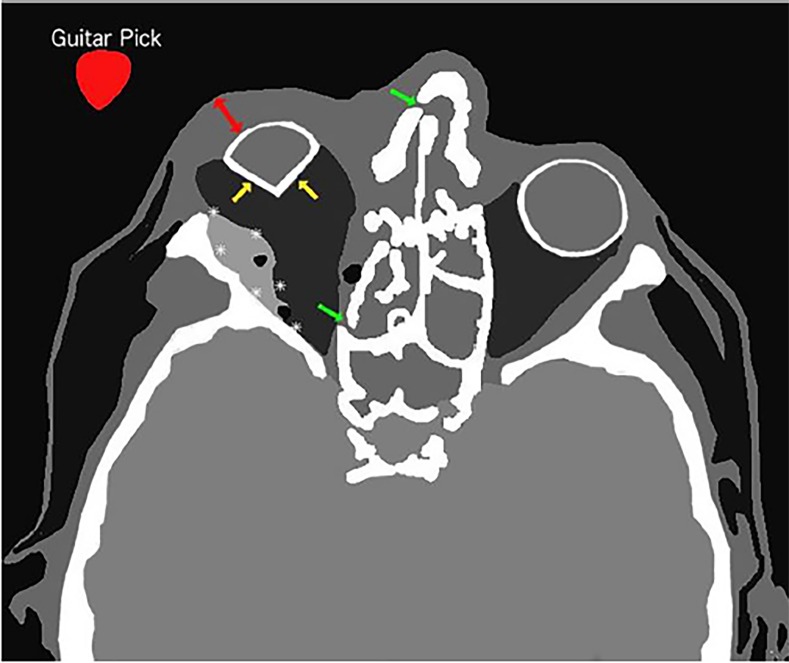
Artistic rendering of computed tomography of facial bones without contrast, axial view, demonstrating retro-orbital hematoma (asterisks), tenting of posterior sclera (also known as “guitar pick” sign) (yellow arrows), eyelid edema (red two-way arrow), bony fractures (green arrows), and superimposed image of a guitar pick (red guitar pick with white text).
